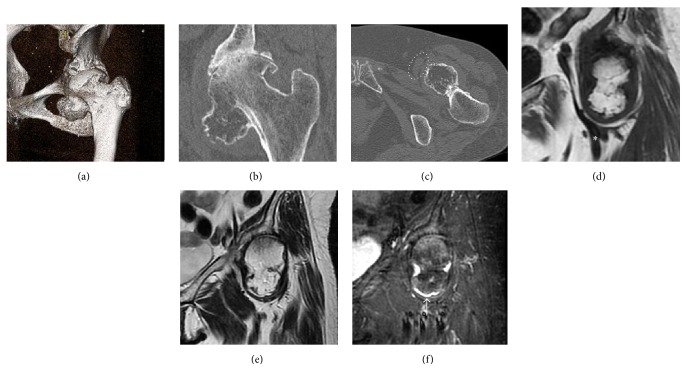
Computed tomography (CT) images and magnetic resonance imaging (MRI) of the left hip. Oblique coronal CT image of the left hip revealed a 40 × 40 × 30 mm sized bone mass connecting to the inferomedial portion of the femoral head. Continuity of the medullary bone is observed at the base of the mass (a, b). The bone mass compressing the femoral vessels is observed on CT (c) and MRI (d); white dotted circle and asterisk indicate the compressed femoral vessels. MRI revealed an intra-articular bone mass surrounded by joint effusion. The intensity of the mass is similar to that of the surrounding normal bone on both T1- (d) and T2-weighted images (e). The cartilage cap corresponding to the very high intensity area is observed at the periphery of the mass on the short-tau inversion recovery (STIR) image (white arrow) (f).
